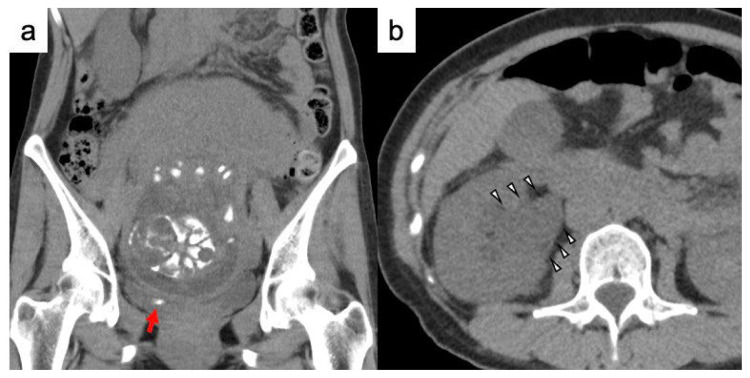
Computed tomography (CT) findings before placement of a ureteral stent a. The red arrow shows a ureteral stone. b. The white arrow heads show hydronephrosis.
